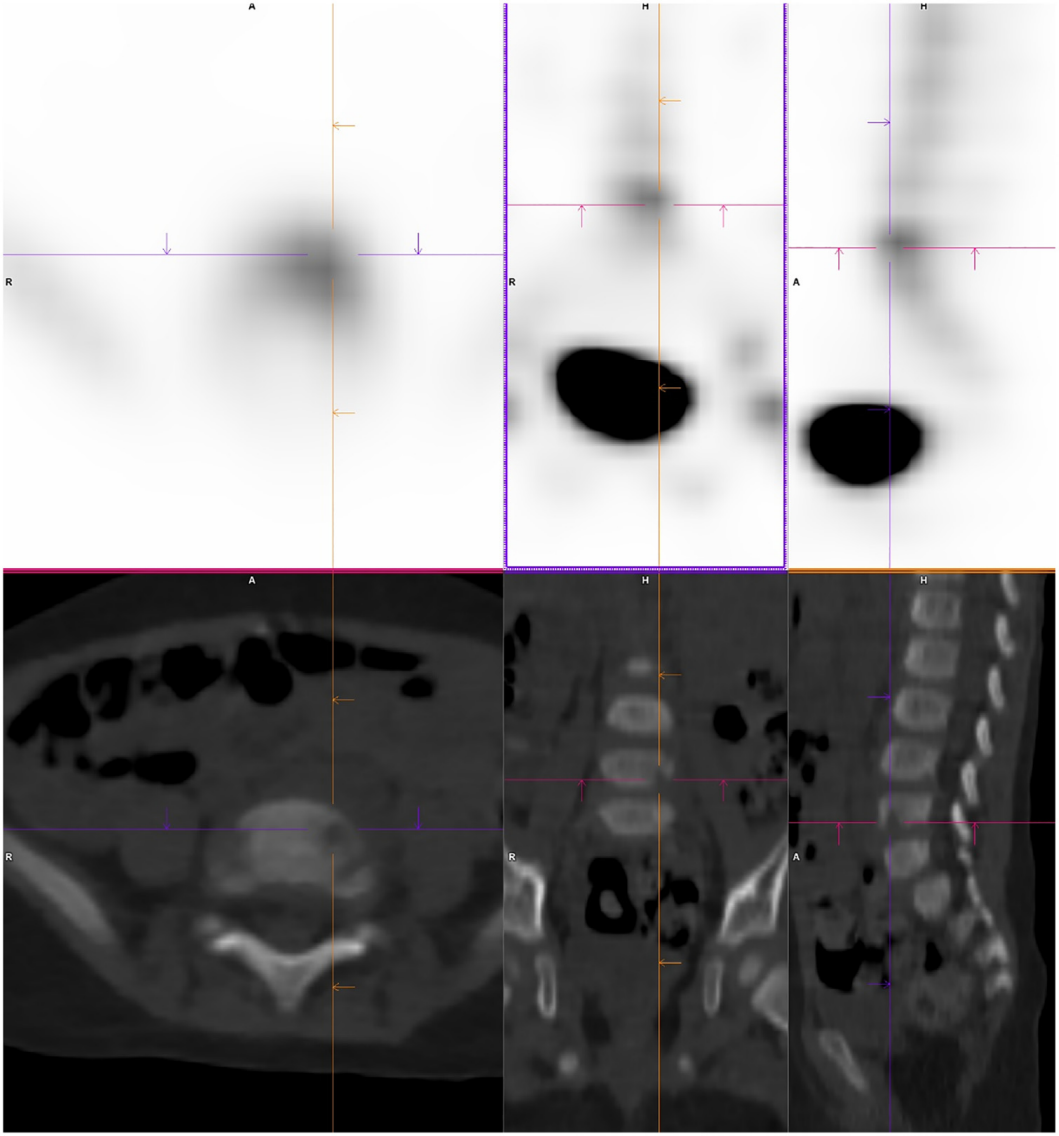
SPECT/CT imaging of the lumbar spine, showing focal increased radiotracer uptake at the site of bony erosion in the left aspect of the L5 vertebral body.
